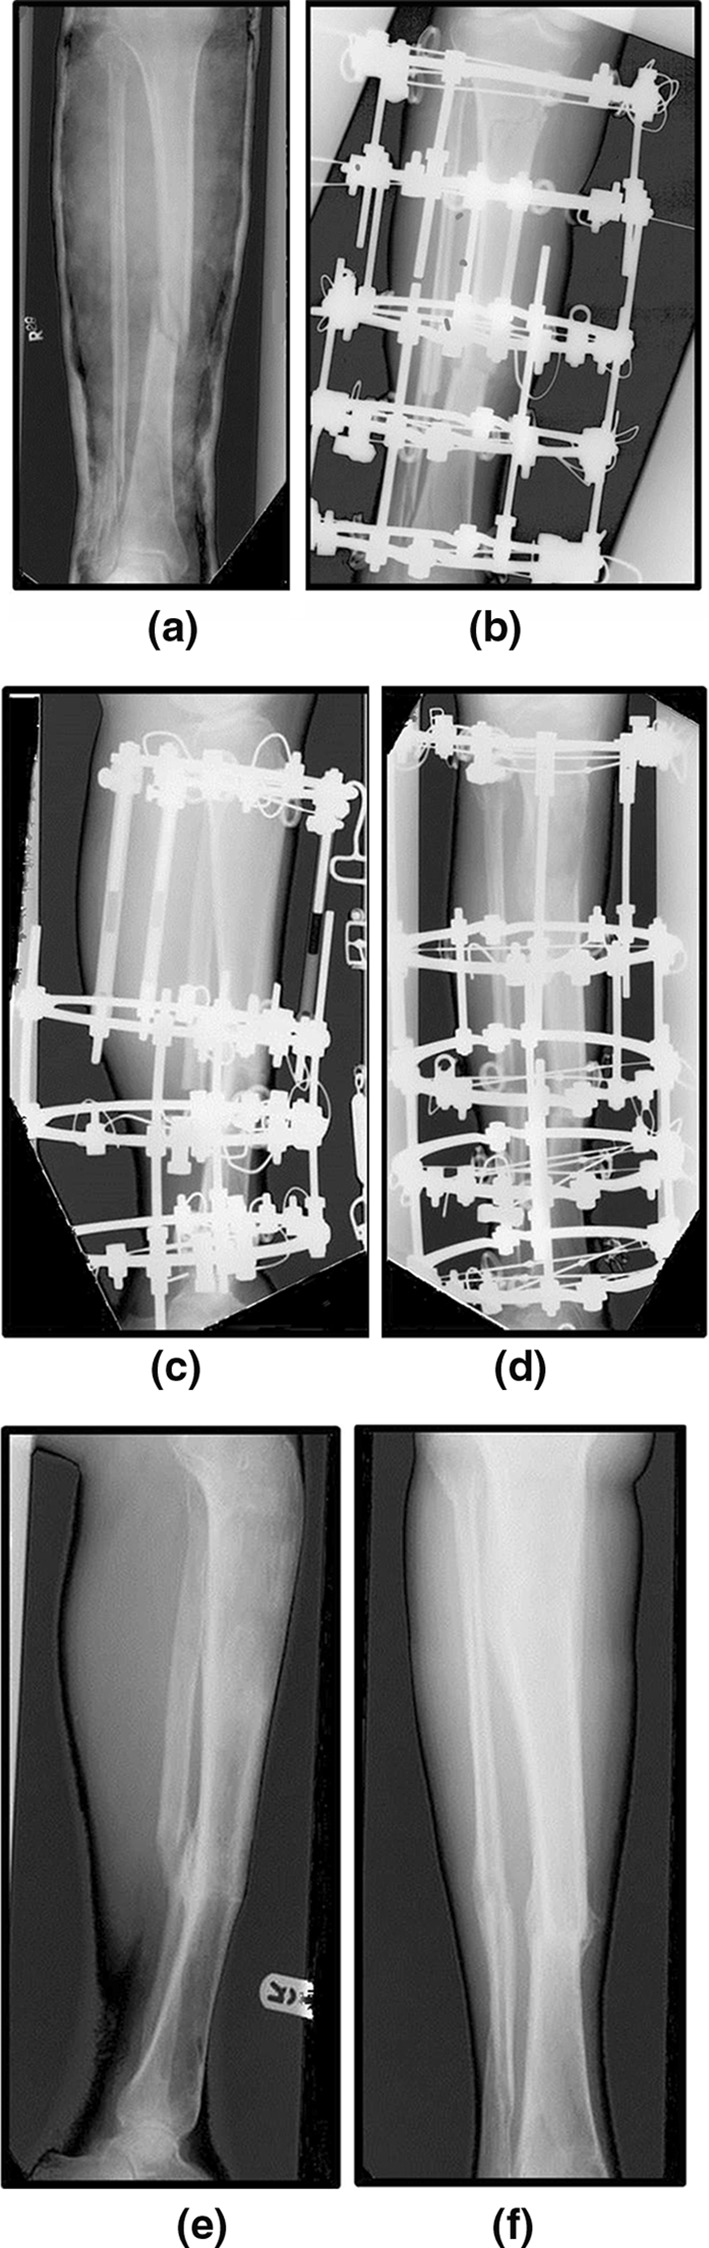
Pre-operative AP radiograph (a). First post-operative radiograph (b) showing proximal corticotomy and frame. AP (c) and lateral (d) radiographs of frame during distraction histogenesis. Final AP (e) and lateral (f) radiographs following frame removal
